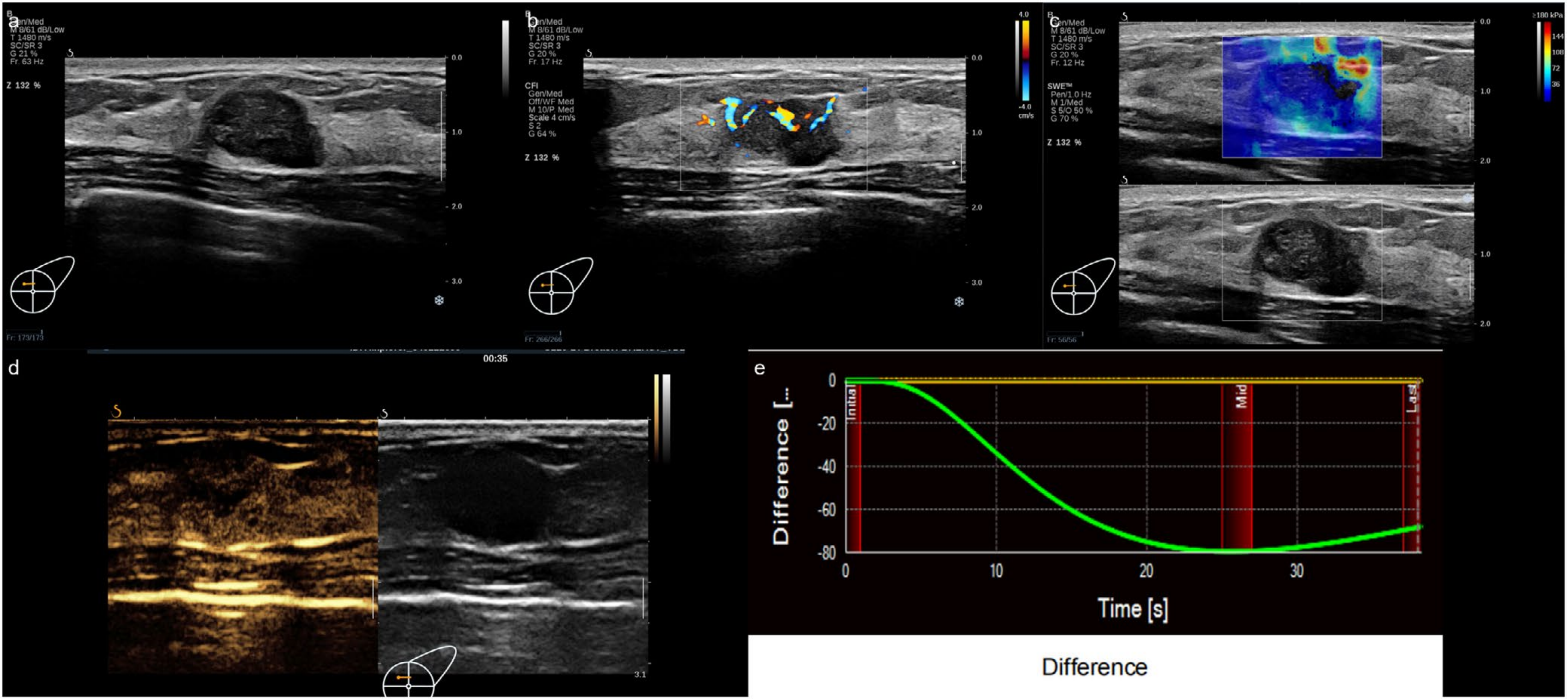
Multi‐modal ultrasound images of HER‐2 negative breast cancer. The lesion presents with irregular shape, enhanced posterior echoes, and Grade III flow. SWE shows a stiff ring sign. CEUS shows low overall enhancement and a negative DVPC wave pattern.
